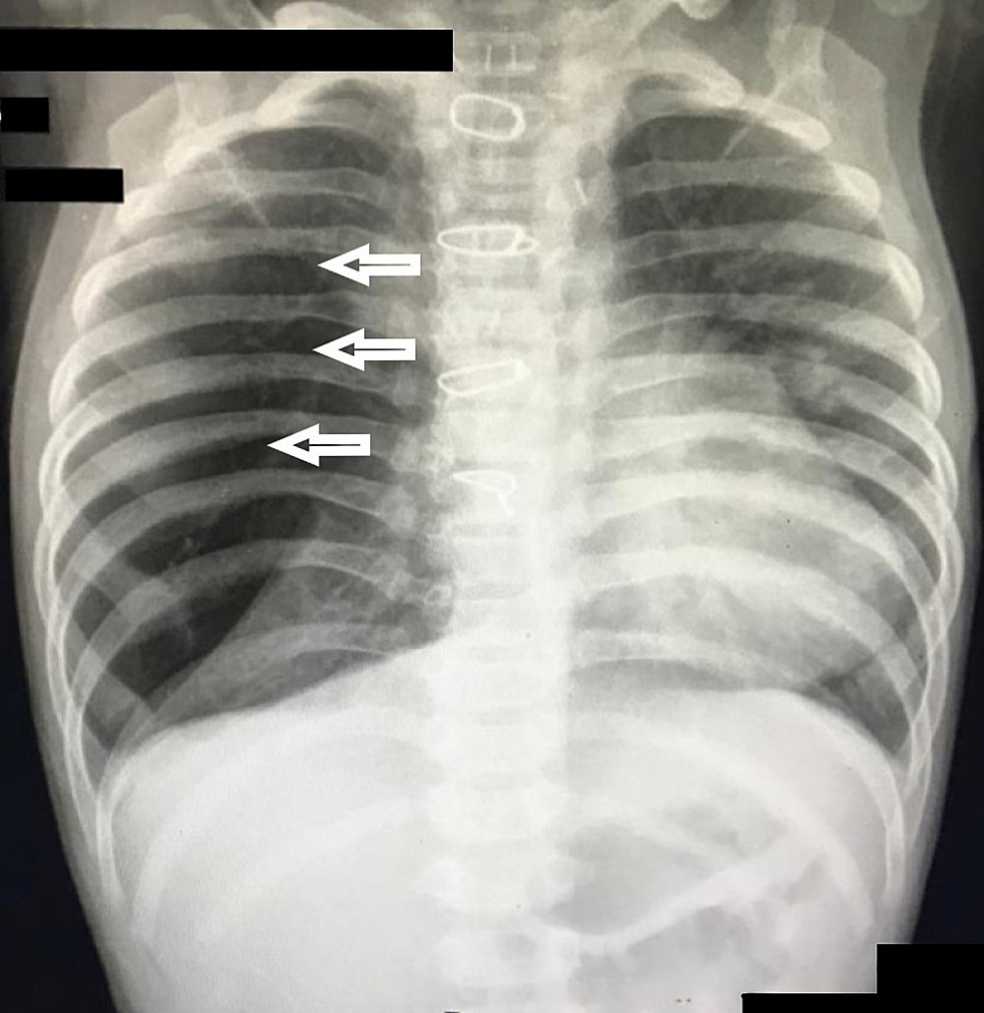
Pre-operative chest X-ray showing right middle and upper zonal hyperlucency (Posteroanterior view)
